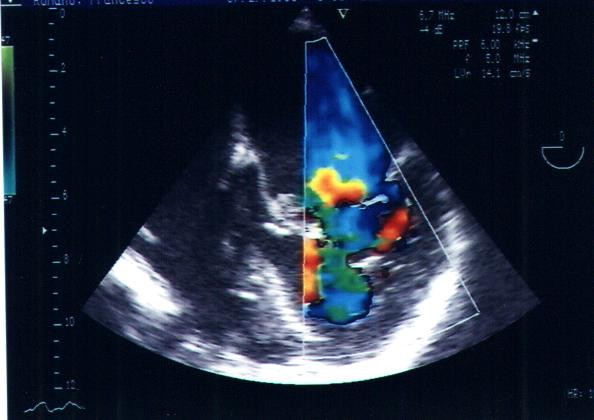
The transthoracic echocardiogram (TTE) showed left ventricular enlargment, atrial dilatation, moderate/severe mitral regurgitation and moderate aortic regurgitation mitral valve masses were suspected.
